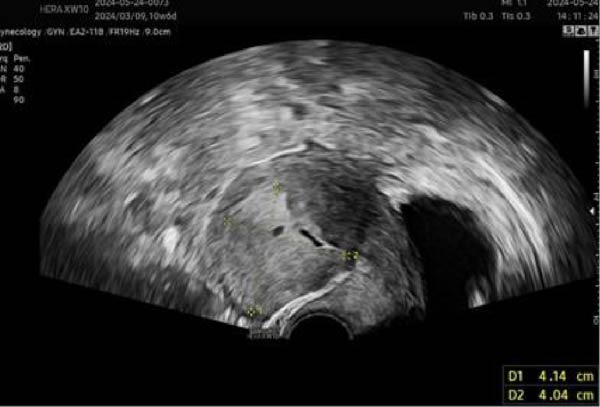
Initial gynecological ultrasound image of Case 2 on May 24, 2024, at our hospital.
